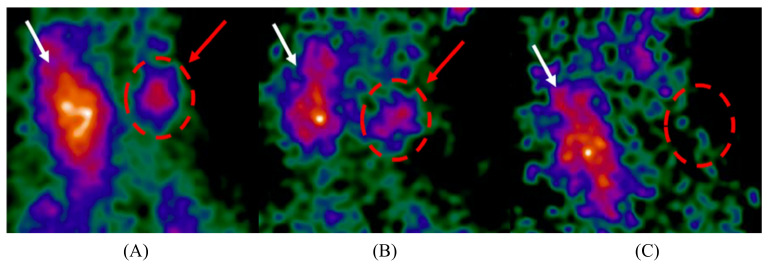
Intraoperative parathyroid scintigraphy images acquired using the Sentinella 102 intraoperative gamma camera. Radiopharmaceutical uptake can be seen in the thyroid gland (white arrow) and a hyperfunctioning parathyroid gland (red arrow and circle). Reproduced from Creighton et al. [4]. (A) Pre-incision image; (B) post-incision image; (C) post-excision image showing the removal of the hyperfunctioning parathyroid gland.
